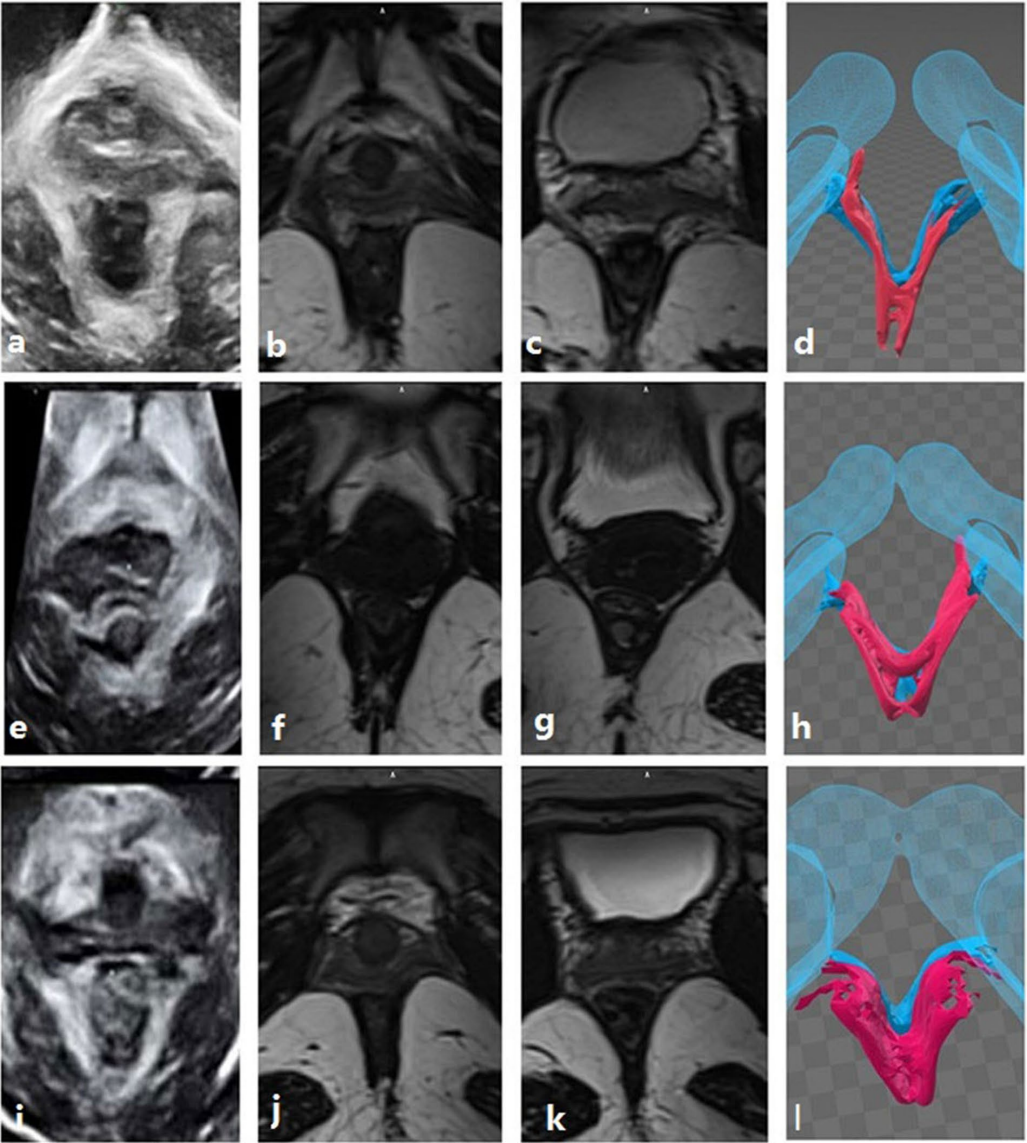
The ultrasound images, MRIs and three-dimensional models of the three cases are shown. (a–l) Represent the same cases, respectively. Images in the first column are ultrasound images, images in the second column are MRIs used to observe the puborectalis, and images in the third column are MRIs used to observe the iliococcygeus and 3D model which show a tomographic overview of the puborectalis and iliococcygeus. Images in the first line are the left puborectalis avulsion with left iliococcygeus avulsion. The images in the second line are the right puborectalis avulsion without iliococcygeus damage. The images in the third line are the bilateral puborectalis avulsion with left iliococcygeus avulsion, including the left internal obturator muscle injury.
